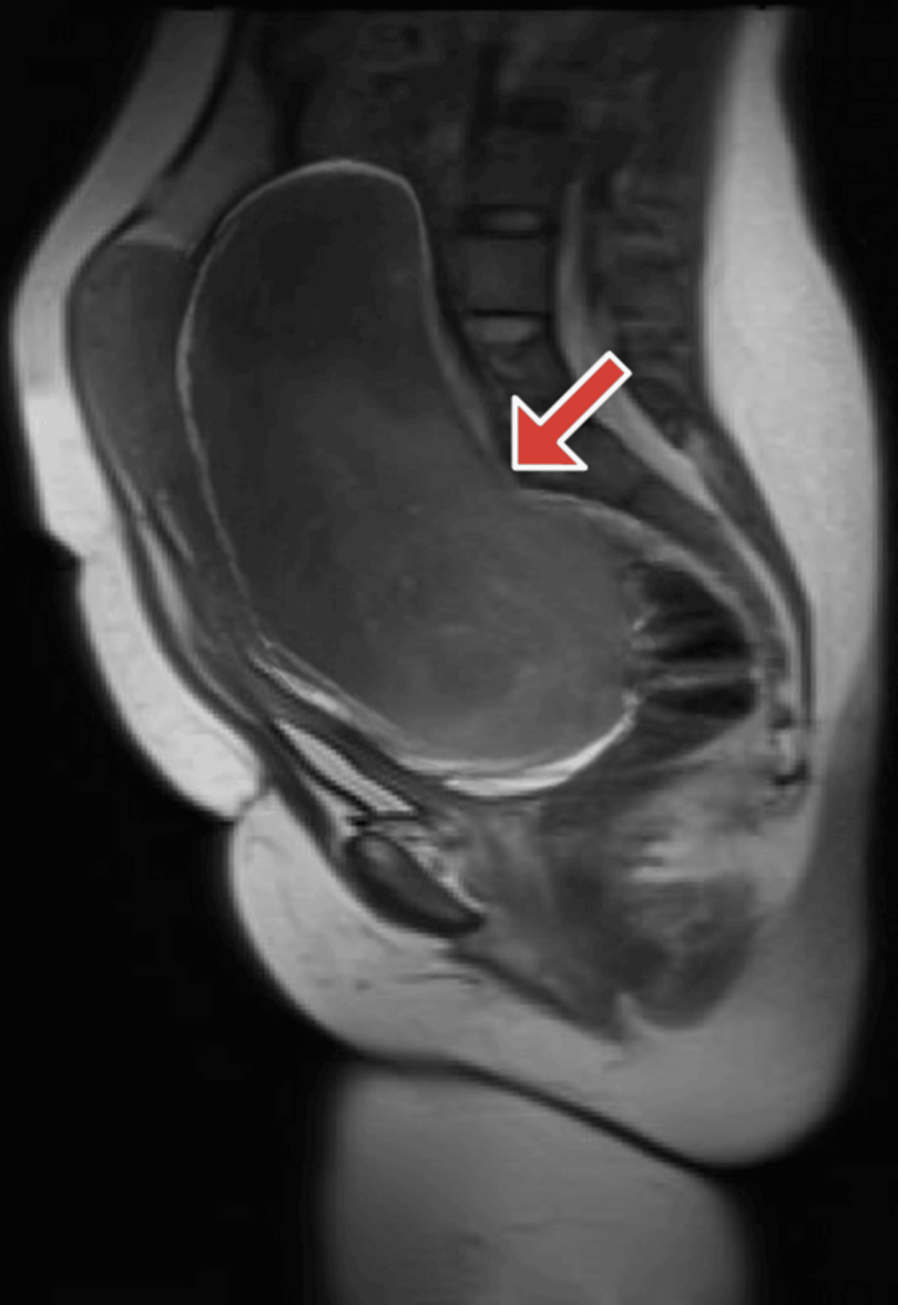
Post-puerperium MRI pelvis with contrast, 10 weeks later, reveals a well-defined oval-shaped pelvic mass compressing the uterus anteriorly and displacing both the rectum and bladder.
